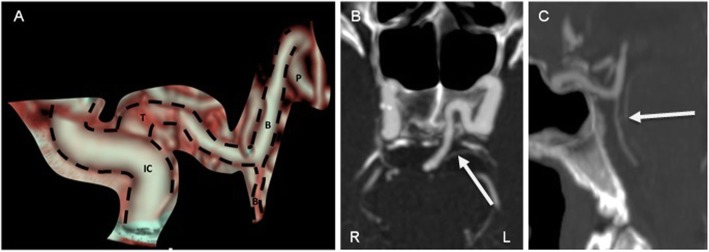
Head CT scan showing the PTA, a) volume rendering technique showing PTA anastomosing the left ICA with de the terminal portion of the BA, b) arrow indicating PTA originating from the left ICA, c) arrow showing hypoplastic BA proximal to the anastomosis. IC – internal carotid artery, T – trigeminal artery, B – basilar artery, P – posterior cerebral artery
